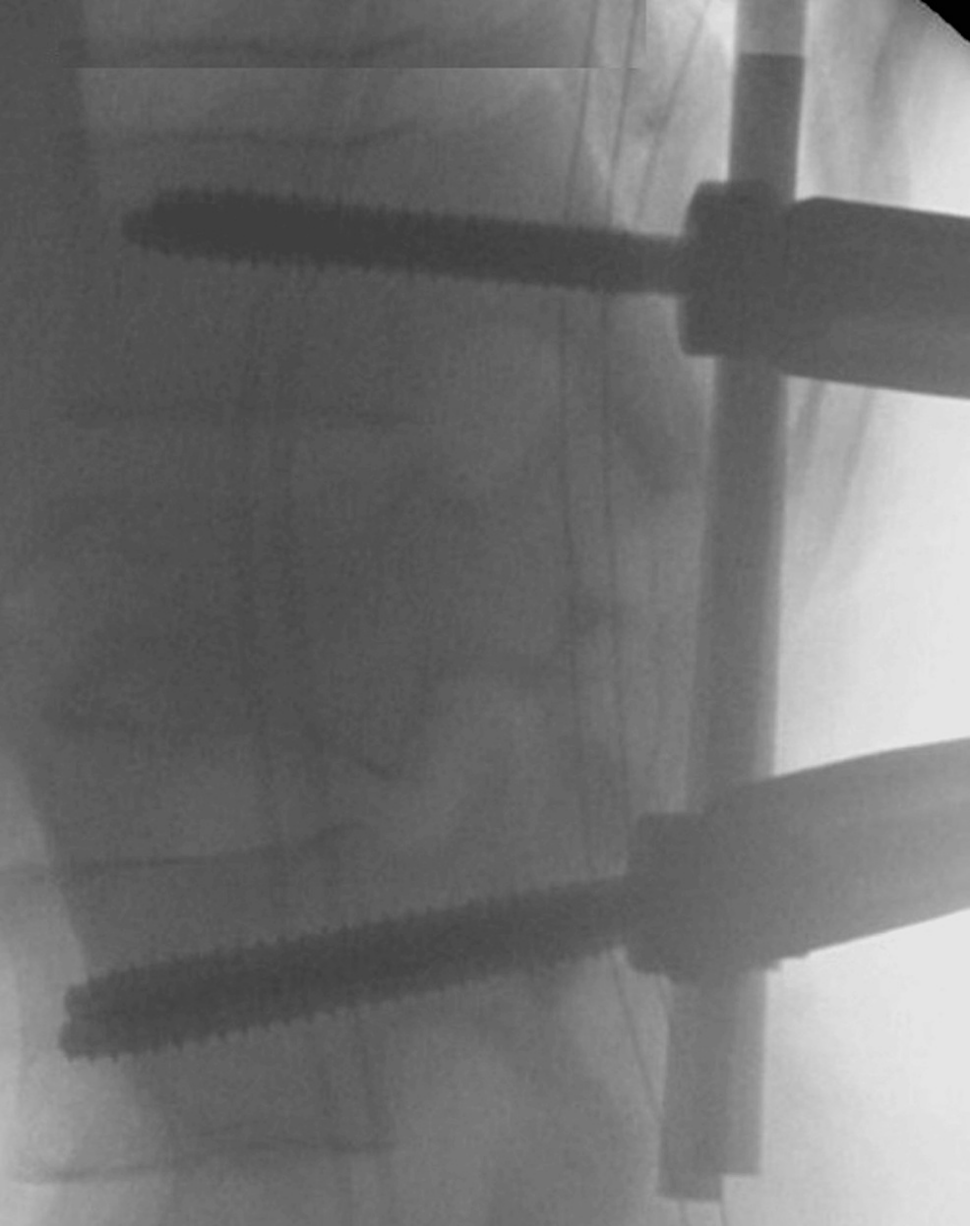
Intraoperative X-rays of minimally invasive fixation
